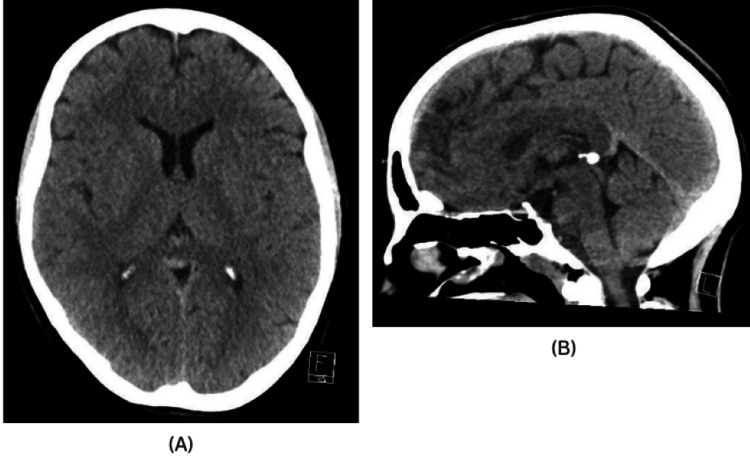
CT of the head. (A) Axial plane. (B) Sagittal plane.
